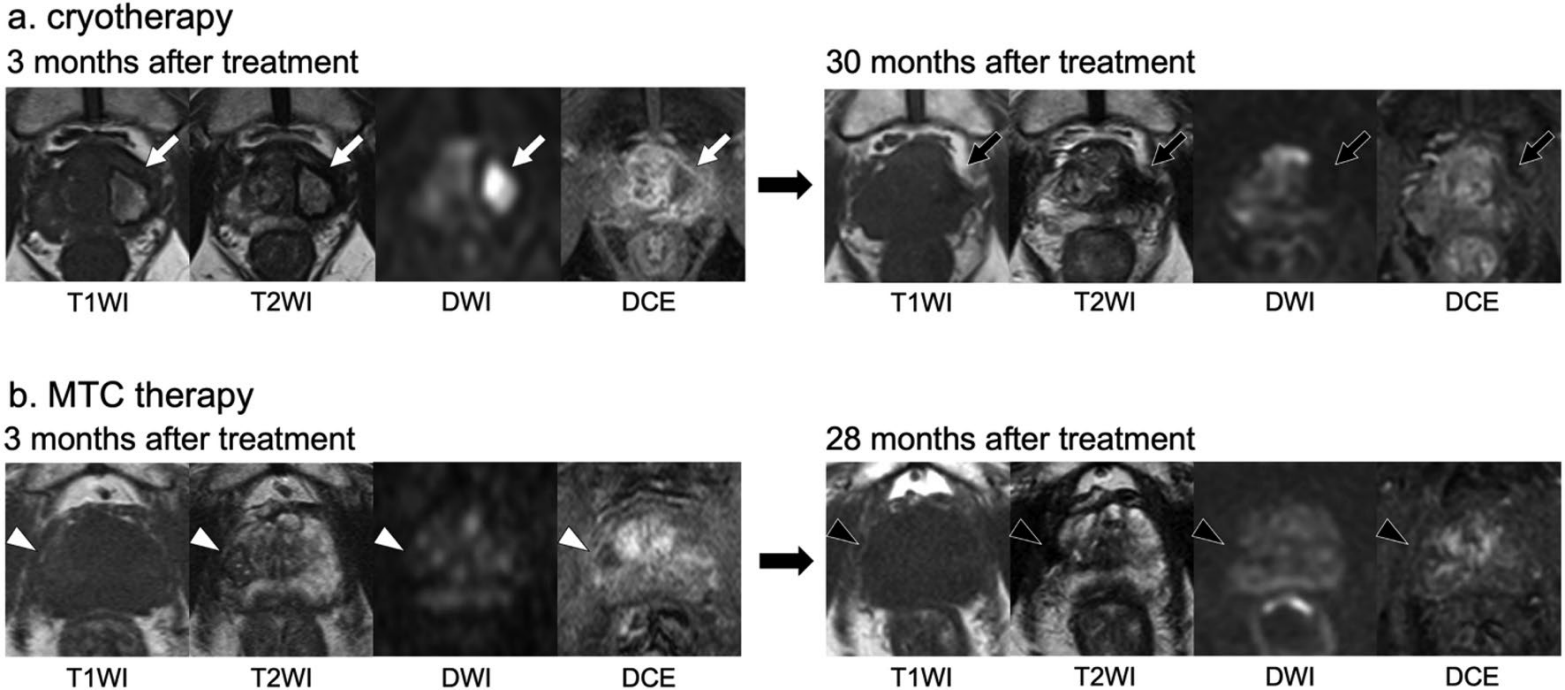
Typical temporal changes in findings on magnetic resonance images after focal cryotherapy and microwave tissue coagulation therapy. a At 3 months after cryotherapy, T1WI showed marked hyperintensity, while T2WI and DWI showed hyperintensity with a hypointense rim. DCE–MRI demonstrated rim enhancement. By 30 months, T1WI, T2WI, and DWI showed hypointensity, and DCE–MRI showed no enhancement. b At 3 months after MTC therapy, T1WI showed slight hyperintensity, T2WI showed heterogeneous hypointensity with a hypointense rim, and DWI showed heterogeneous mixed signal intensity. DCE–MRI showed rim enhancement. A small spot of high T2 signal within the treated area corresponds to the needle tract. By 28 months, T1WI, T2WI, and DWI showed hypointensity, and DCE–MRI showed no enhancement. Although early post-treatment MRI findings differed between the two therapies, late-stage findings were similar. *DCE–MRI* dynamic contrast-enhanced magnetic resonance imaging, *DWI* diffusion-weighted imaging, *MTC* microwave tissue coagulation, *T1WI* T1-weighted imaging, *T2WI* T2-weighted imaging
